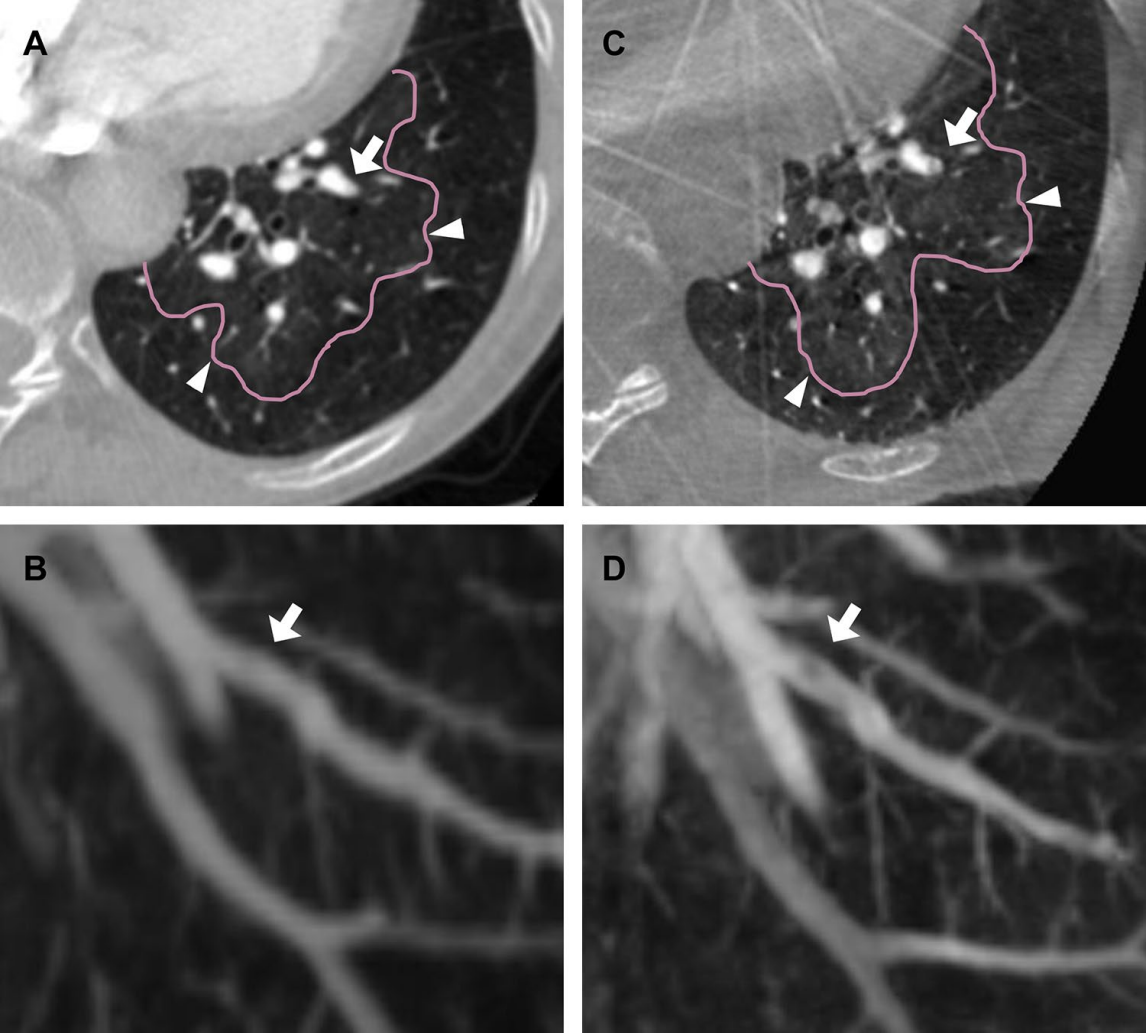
Depiction of peripheral intravascular lesions on CT and CACT. (A, C) axial multiplanal refomation of the left lower lobe shows mosaic perfusion (arrow heads) on CTPA (A) and CACT (C). (B, D) corresponding 20 mm maximal intensity projection of CTPA (B) and CACT (D) reveals an intravscular band in pulmonary artery segment VIII (arrow) as a typical finding for CTEPH on CACT (D) but not on CTPA (B).
